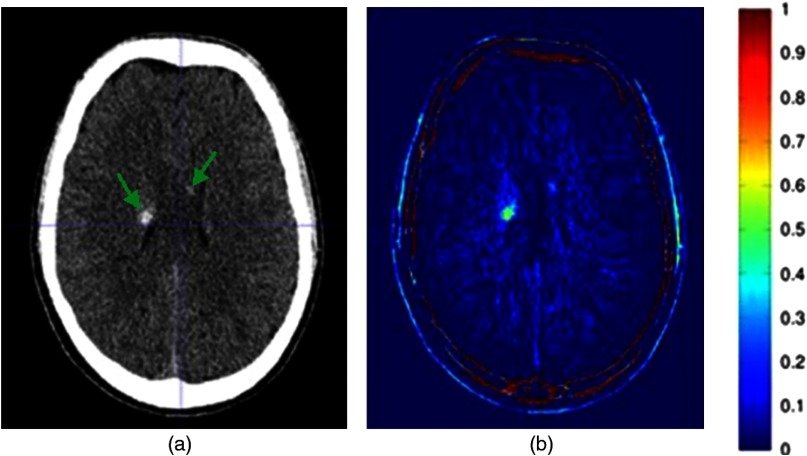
(a) A slice of the original CT image of a TBI patient with two small regions of hemorrhage (green arrows). (b) After applying the automatic detection method on the original image, the two hemorrhages were easily distinguishable from the healthy tissue. However, high intensities were also detected at the edges of the skull.
